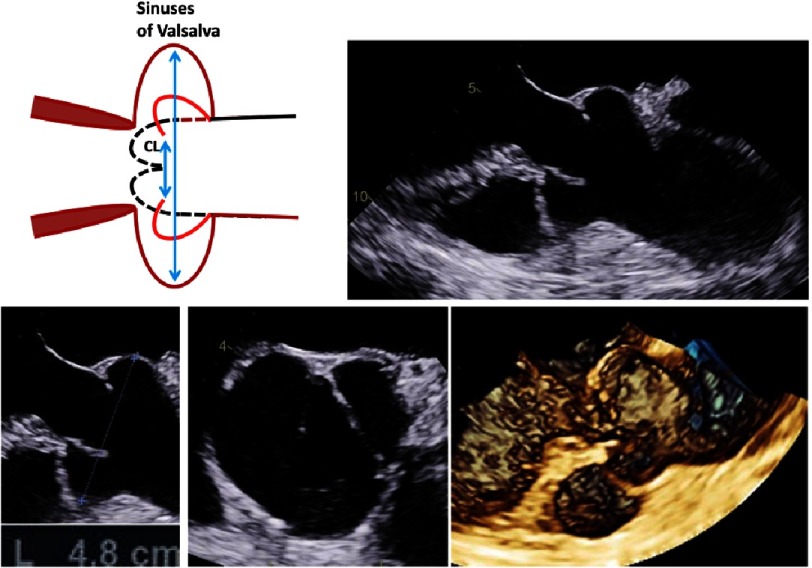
The scheme shows an isolated aneurysm of the sinuses of Valsalva. On the right side and below 2D and 3D transesophageal images during systole as well as the measurement of the aortic root diameter are shown.
